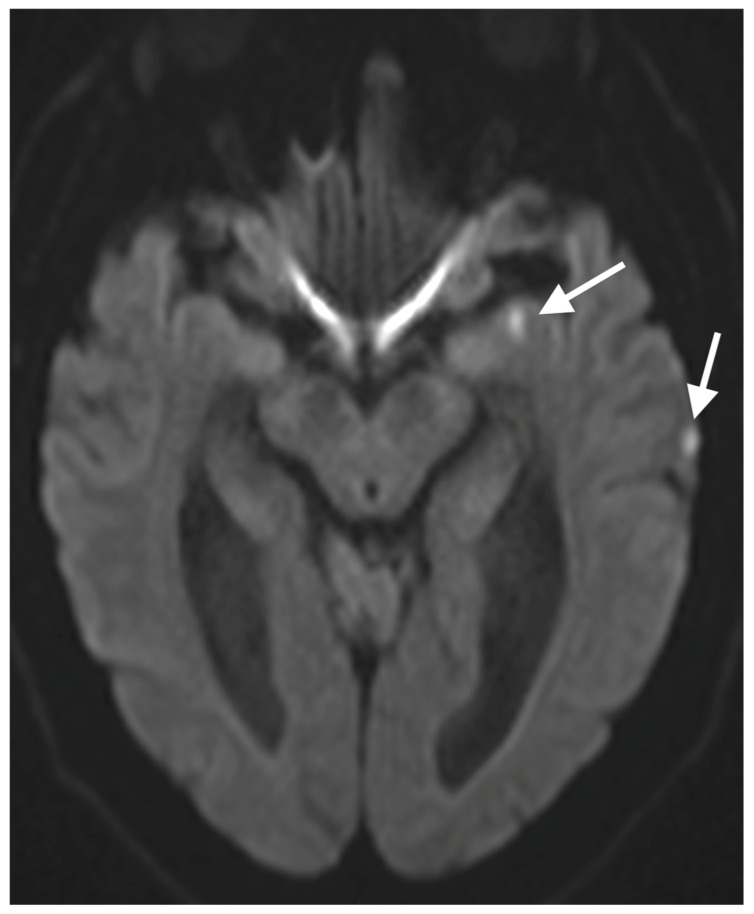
The Brain DWI MRI 24 h after the initial ischemic attack.
